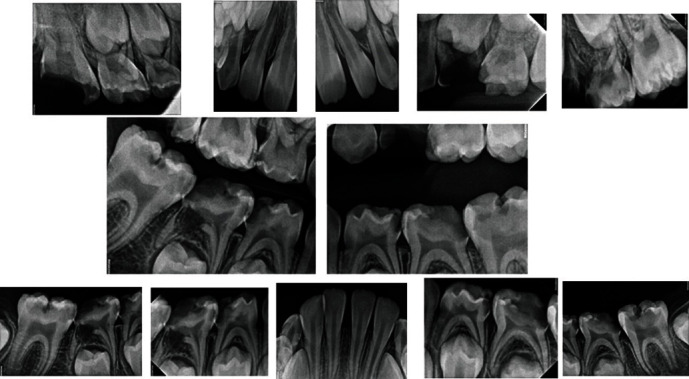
Full mouth preoperative radiographs.
